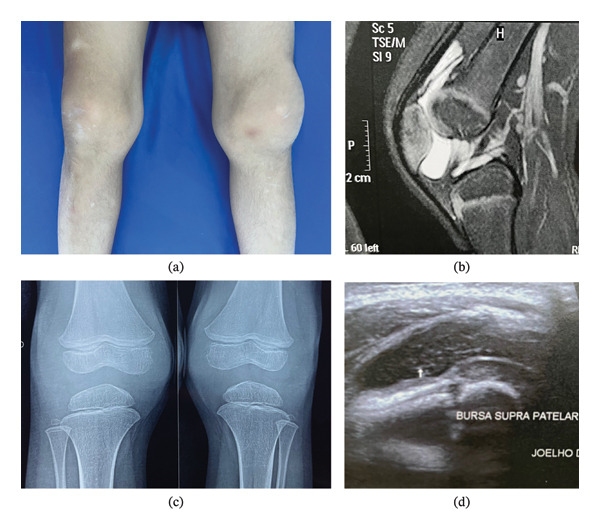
Patient 1: (a) voluminous bilateral knee swelling; (b) MRI of the right knee showing moderate joint effusion; (c) X‐ray showing increased joint space in the knees; (d) knee ultrasound showing debris in the suprapatellar bursa.
